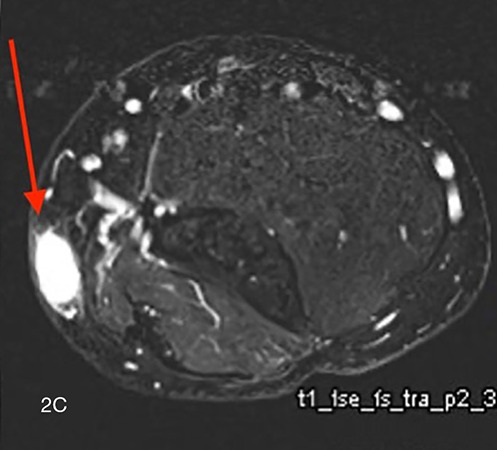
Magnetic Resonance Imaging shows an oval‑shaped, well‑defined nodule in the subcutaneous tissue. A. The lesion appears isointense to muscle on T1‑weighted images (arrow) and B. is hyperintense on fatsuppressed T2‑weighted images (arrow). C. After intravenous administration of gadolinium contrast, there is vivid, homogeneous enhancement of the lesion (arrow), in keeping with a vascular tumor.
